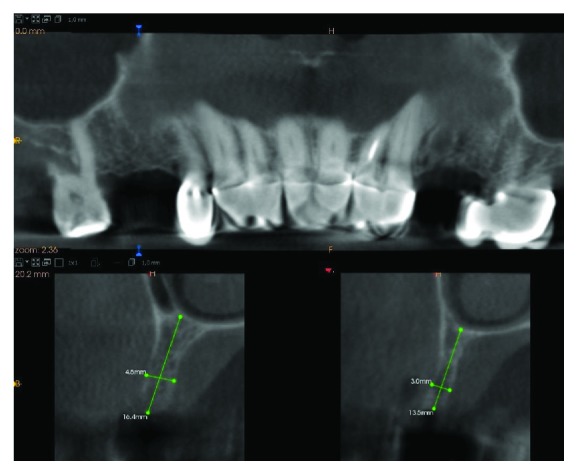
A CBCT scan of the patient with a very thin maxillary alveolar ridge.
